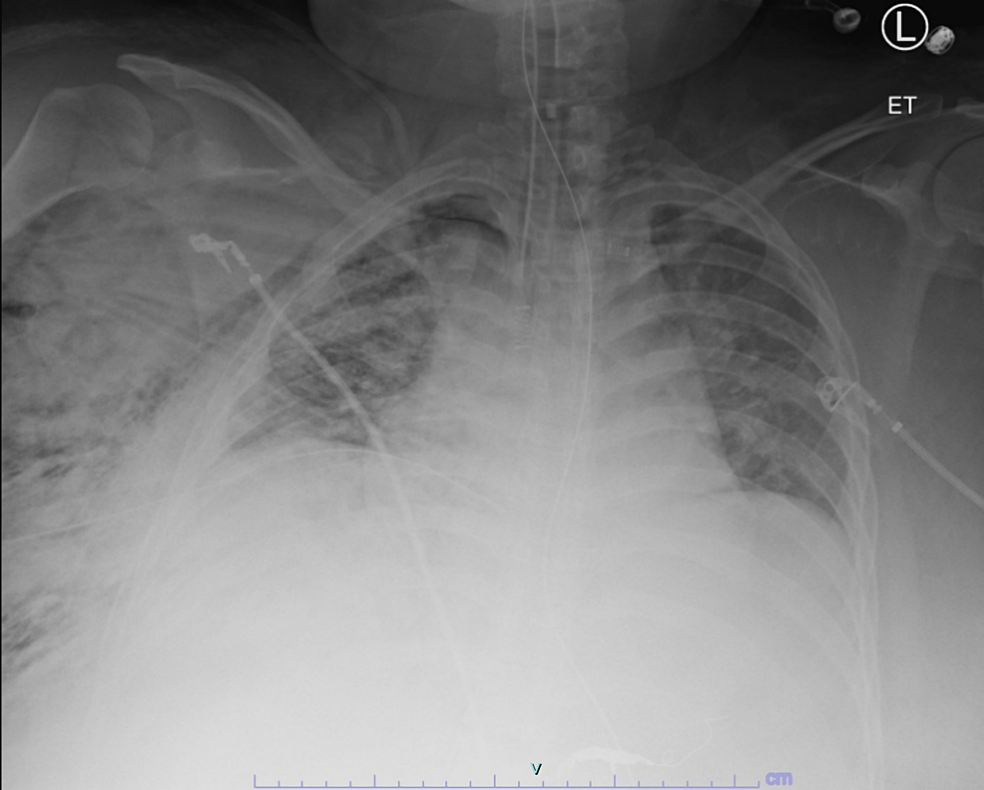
Portable chest X-ray taken immediately after tube thoracostomy shows the extent of the right-sided subcutaneous emphysema.
